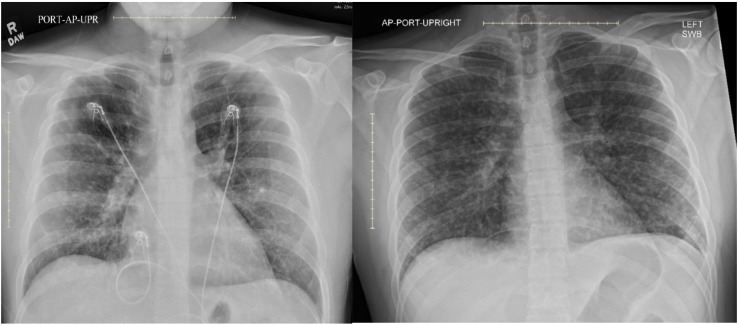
Patient’s chest radiography findings on admission (left) and several days after (right), revealing progressive diffuse reticulonodular opacities.
